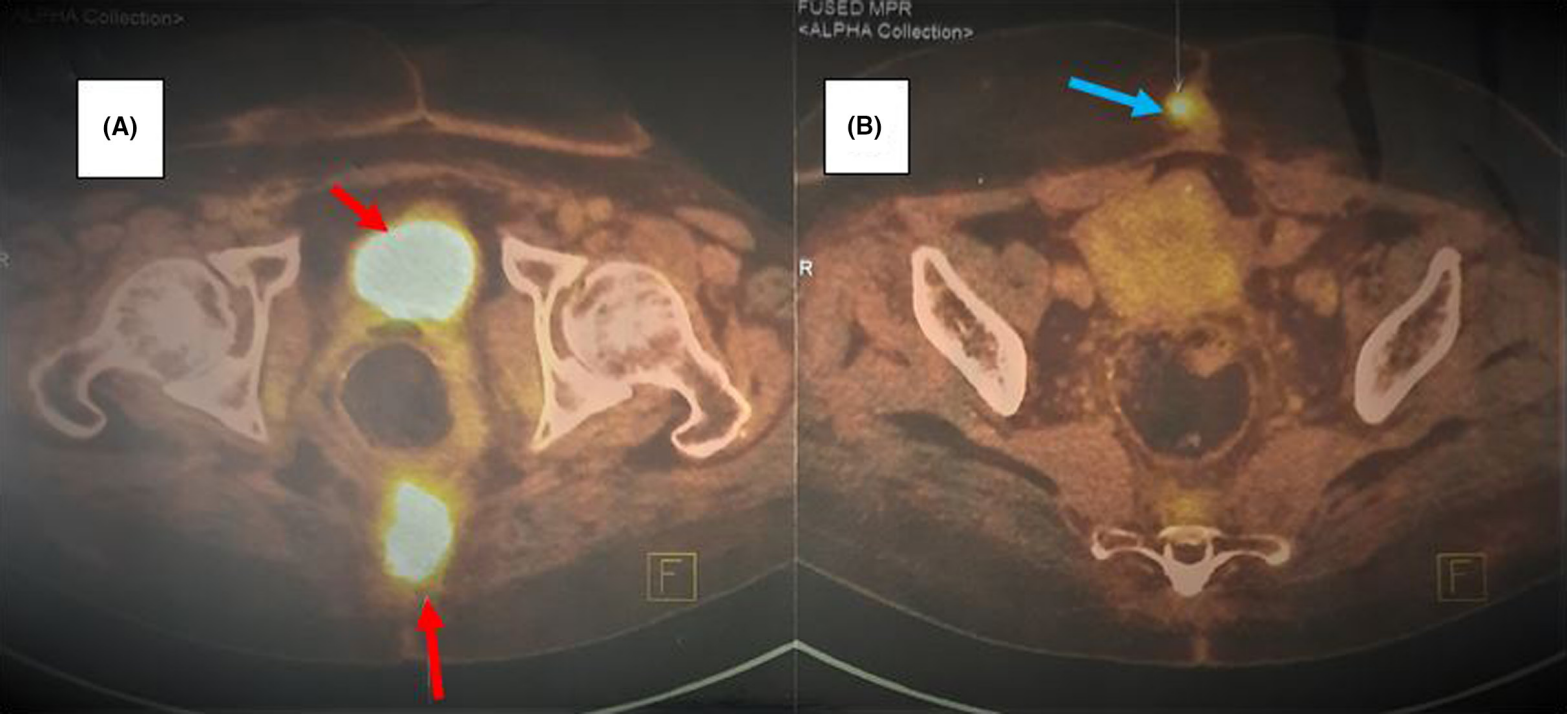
FDG‐PET/CT scan (A) There is an FDG avid (SUVmax = 17.0) soft tissue mass in the pre‐coccygeal region (arrows) measuring 3.8 × 3.0 cm. (B) There is an FDG avid (SUVmax = 6.3) soft tissue lesion in anterior pelvic wall (blue arrow) measuring 1.3 × 1.2 cm reveals metastatic lesion.
